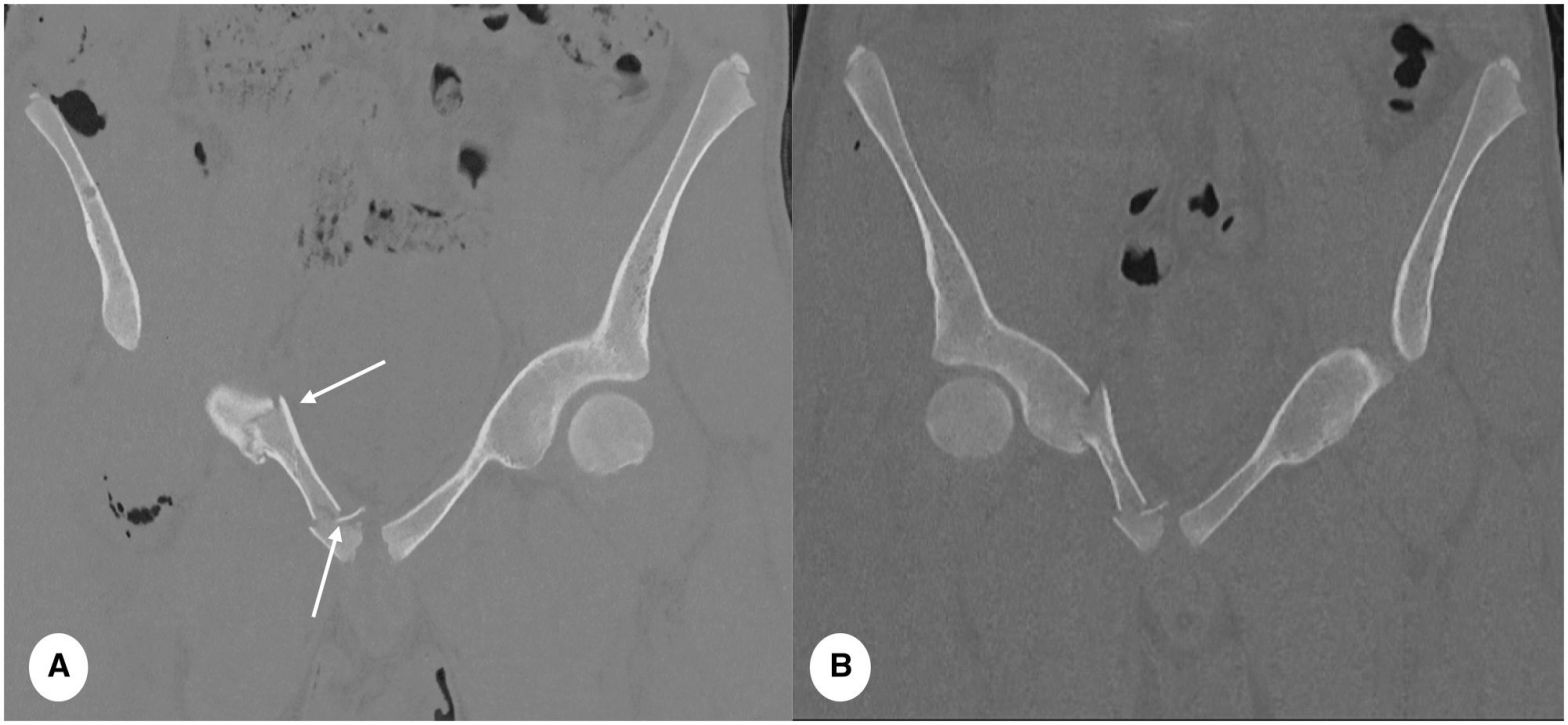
Comparison of hip fracture assessment between PCD-CT and EID-CT in 16 year old male after pelvic trauma. (A) PCD-CT (slice thickness 1 mm, reconstruction kernel Br68 and IR level 3, Q3) and (B) EID-CT (slice thickness 1 mm, reconstruction kernel l70h and IR 3) on the same subject. Note higher SNR and clearer definition of cortical bone fracture fragments (thin arrows) in (A) and superior pubic ramus fracture (thick arrow). Bone contours in (B) are less sharp with reduced signal of cortical bone.
